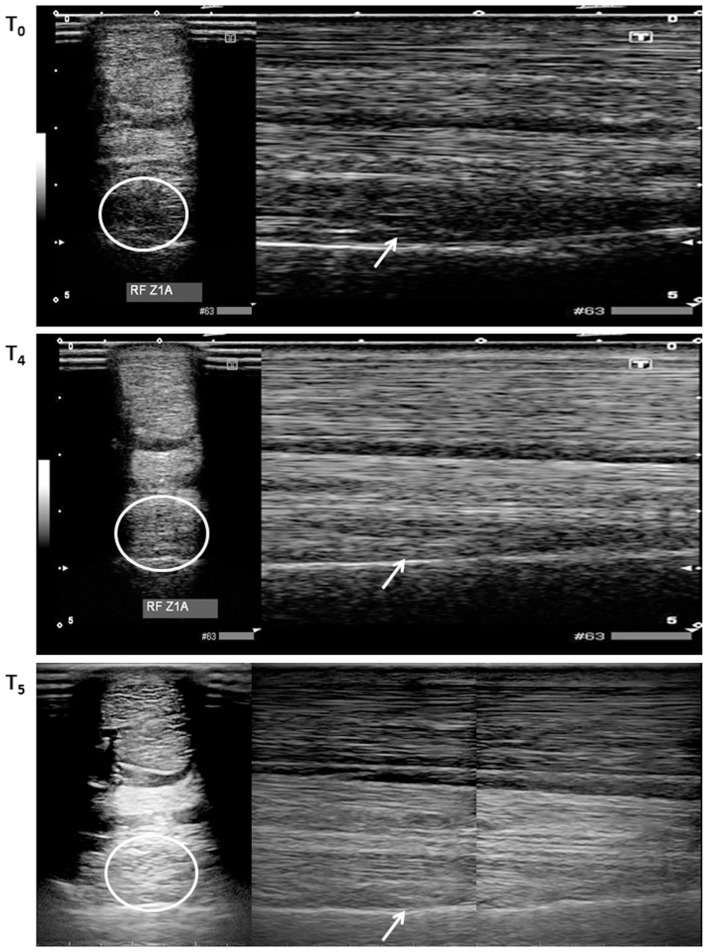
Transverse (encircled lesion area) and longitudinal (arrow in lesion area) ultrasound images of the proximal aspect of the lateral lobe of the suspensory ligament performed at *T*_0_, *T*_4_ (12 weeks), and *T*_5_ (16 weeks). At *T*_0_, a clear hypo-echoic area could be noticed on both ultrasounds. At *T*_4_, only limited remaining hypo-echoic areas were noticed, whereas this clearly reduced at *T*_5_.
